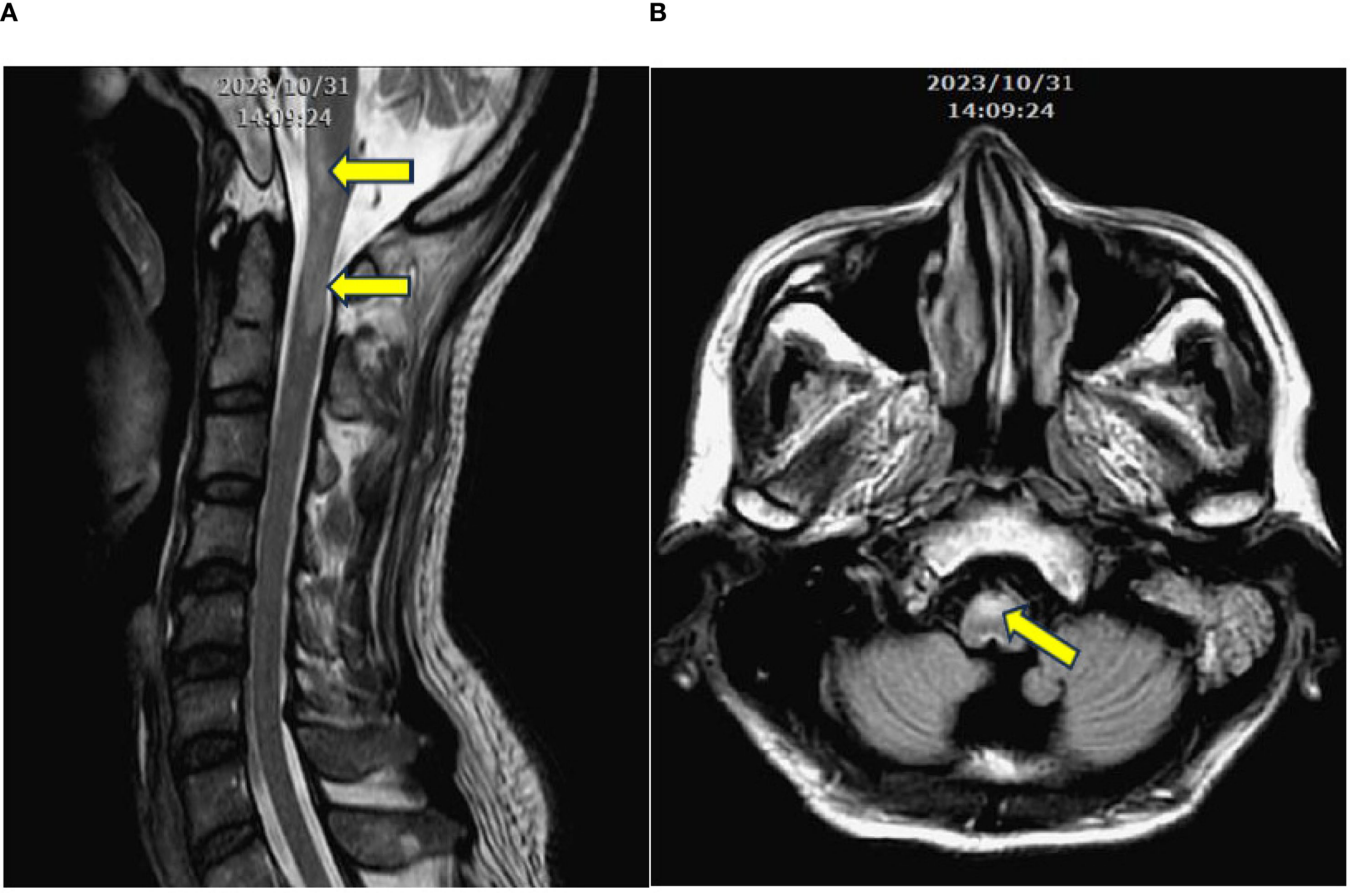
(A) Contrast-enhanced T2-weighted MRI in the longitudinal view, revealing a longitudinal signal increase extending from the level of medulla to C1. (B) Signal increase at the anterior portion of the medulla (arrows).
